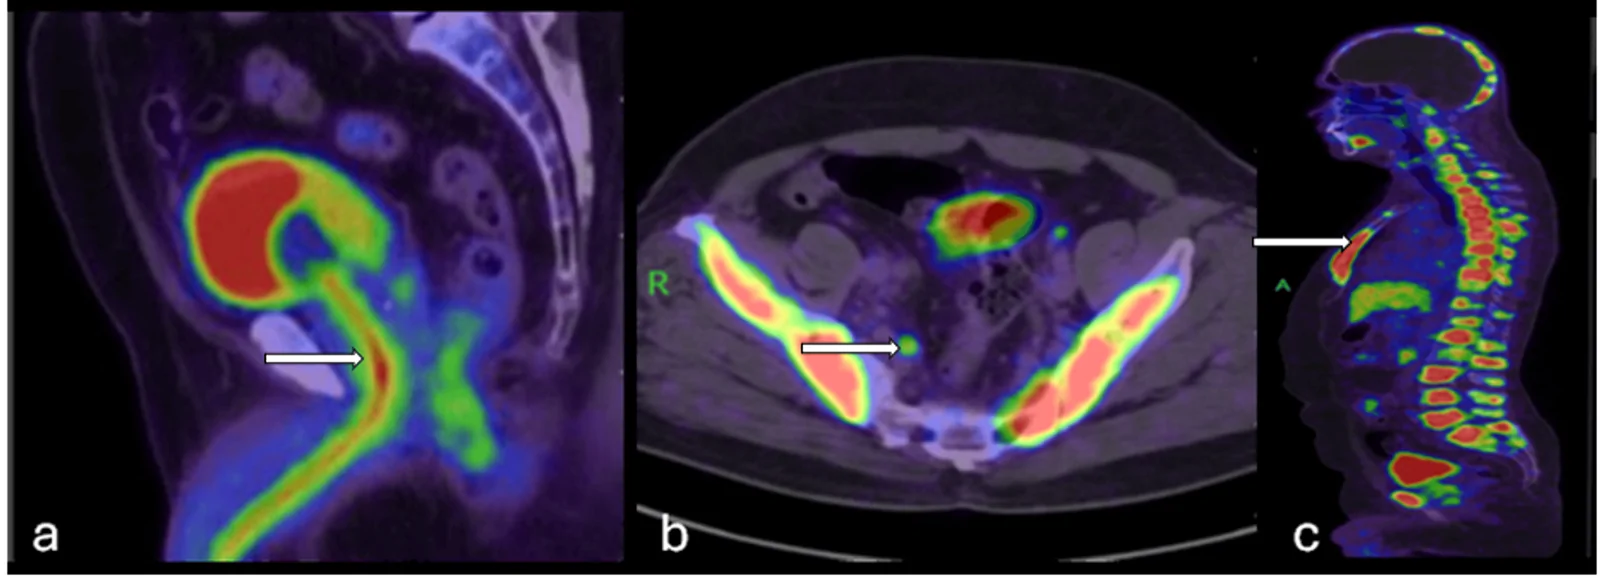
PSMA PET-CT findings during the patient’s clinical course. (a) Baseline sagittal image showing an enlarged prostate measuring 47 × 50 × 21 mm with mild intravesical extension (white arrow). (b) Axial image showing PSMA-avid right internal iliac and left external iliac lymph nodes, with additional non-PSMA-avid right obturator and external iliac nodes (white arrow). (c) Whole-body sagittal image showing multiple PSMA-avid lesions noted in the skull, spine, ribs, pelvis, scapula, clavicle, and sternum, likely metastases (white arrow). PSMA = prostate-specific membrane antigen; PET-CT = positron emission tomography-computed tomography
